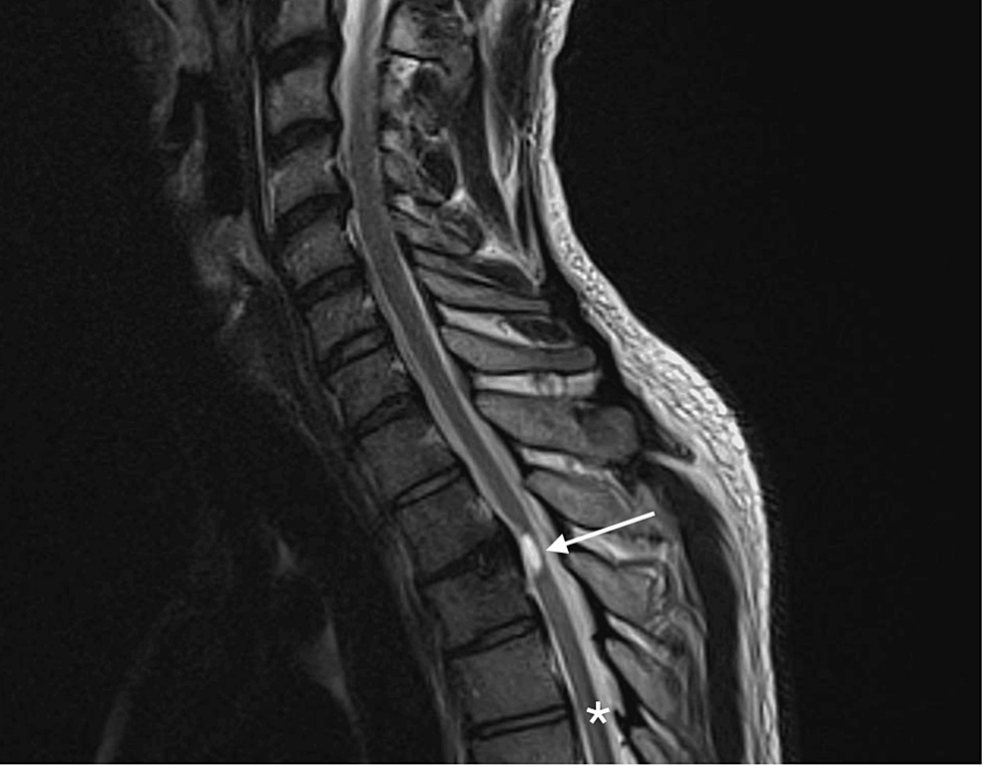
Case 2: MRI of the spine Stable syrinx and parenchymal volume loss around the syrinx at T2-T3 (arrowed). Superficial siderosis is partially visualised at T4-T5 (star).
